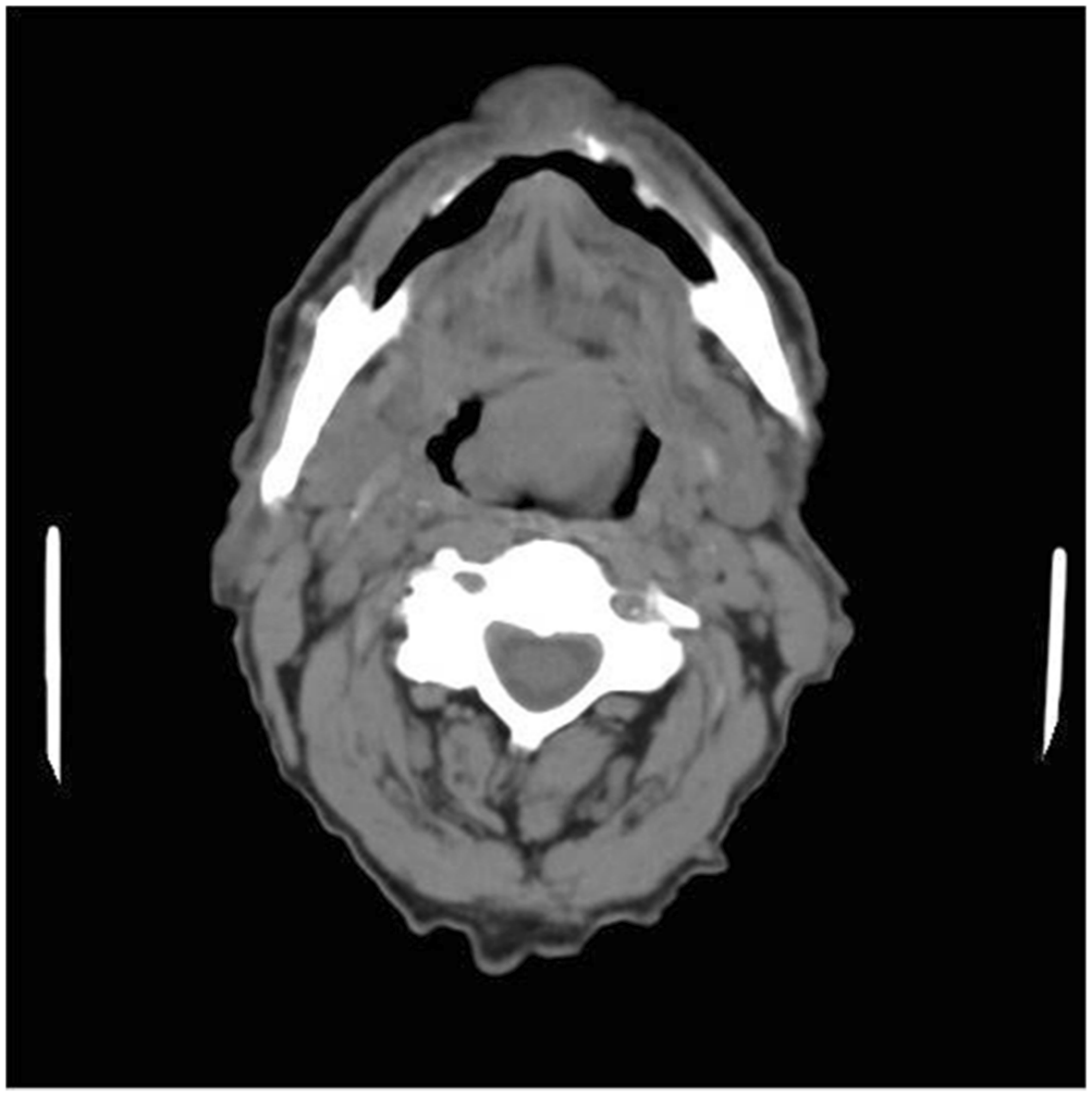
Computed tomography image demonstrating the mass.
